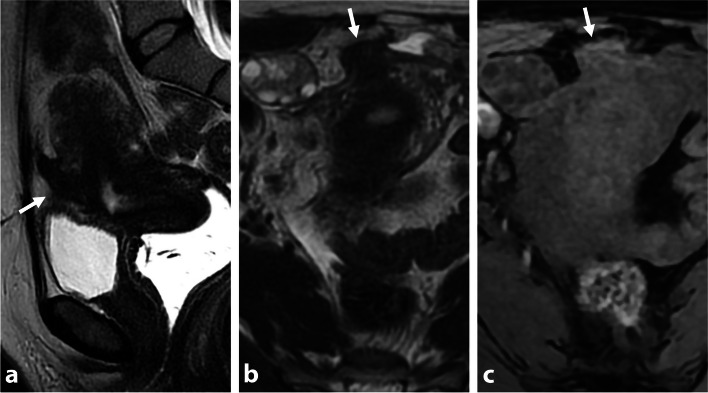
Anterior adhesions in the vesicouterine pouch after C-section in a 40-year-old woman with medical history of pelvic endometriosis. a Sagittal and (b) axial T2-W MR images show a T2-hypointense fibrous thickening of the vesicouterine pouch, with severe adhesions of the uterine body (arrows). c Axial T1-W fat-suppressed MR image shows no hemorrhagic foci in the anterior subperitoneal space (arrow). Endometriosis surgical management has been decided. During surgical procedure, pelvic anterior symphysis was proven with no obvious endometriosis lesion (confirmed with negative biopsies at pathology)
